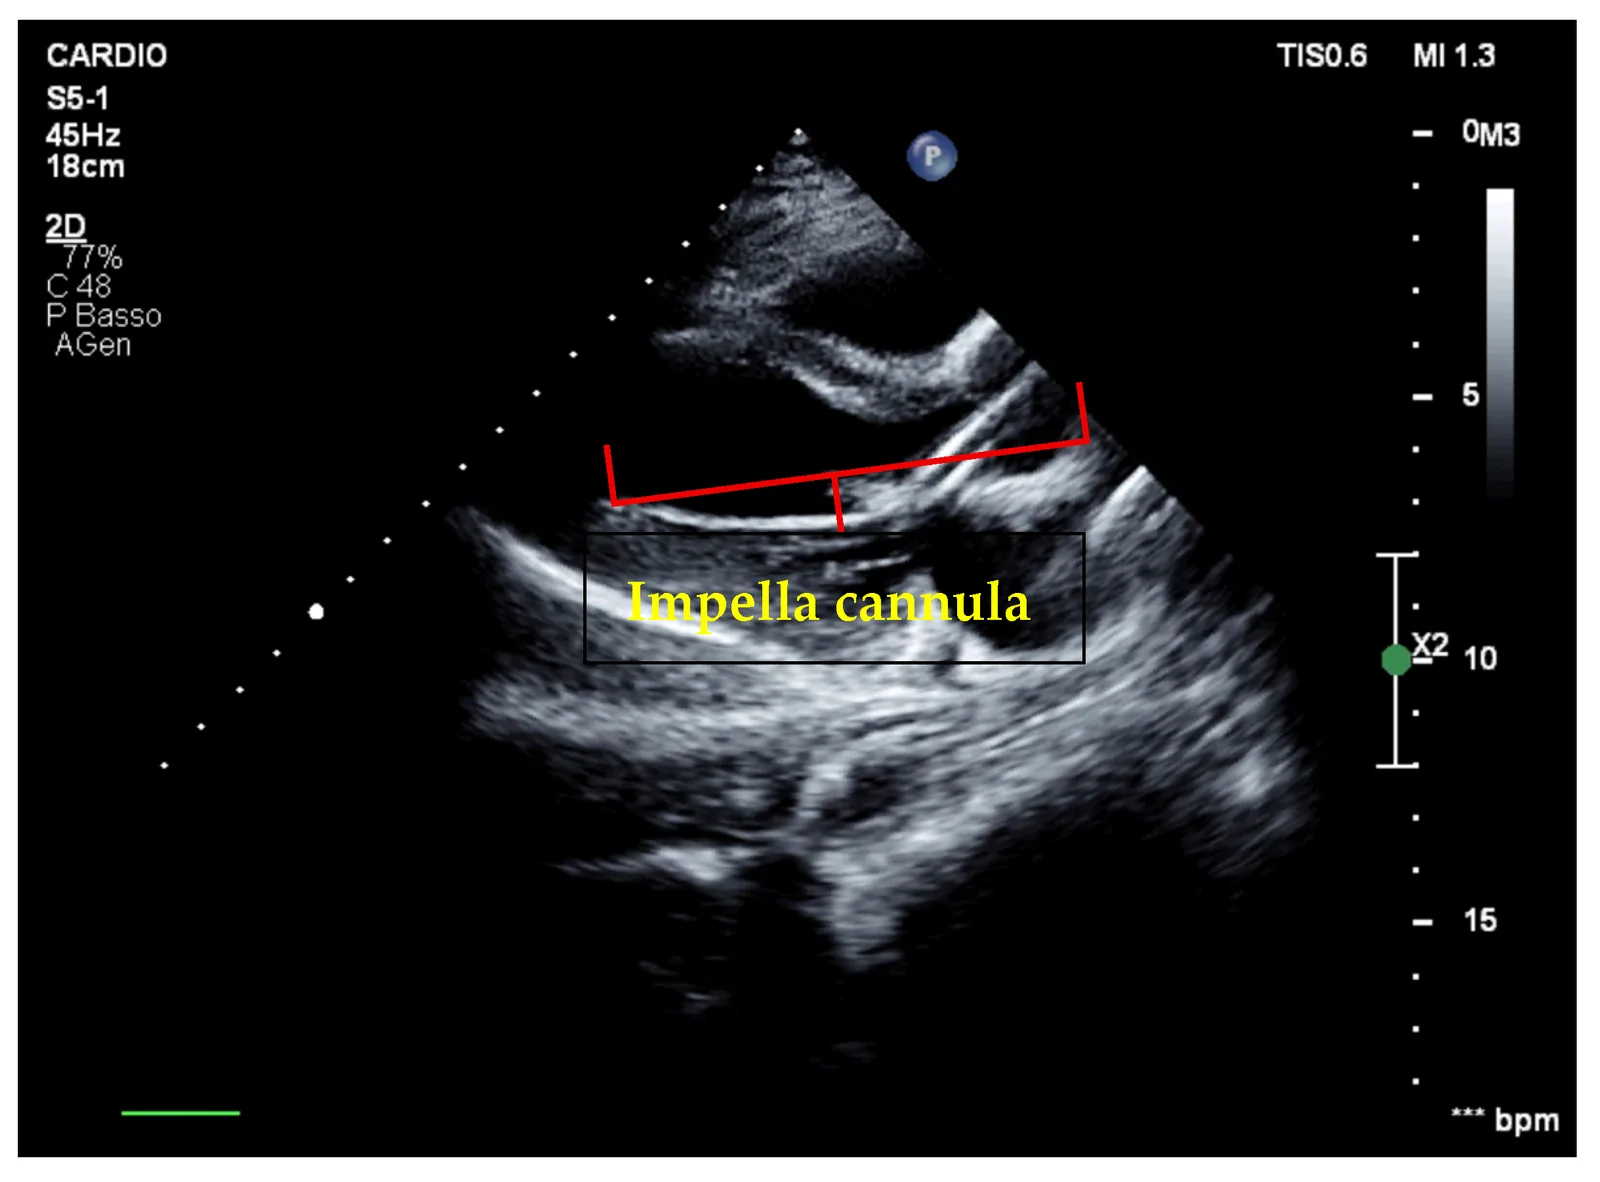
Echocardiography parasternal long-axis view showing the correct positioning of the IMPELLA system.
